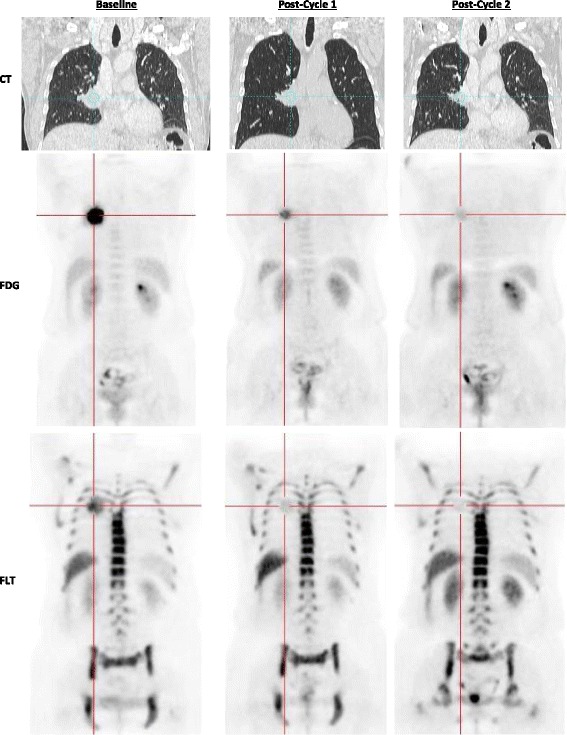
Representative images of a patient (patient 2) classified as a “responder”. CT, FDG, and FLT images are shown at each indicated time point. The same lesion is targeted in each image
